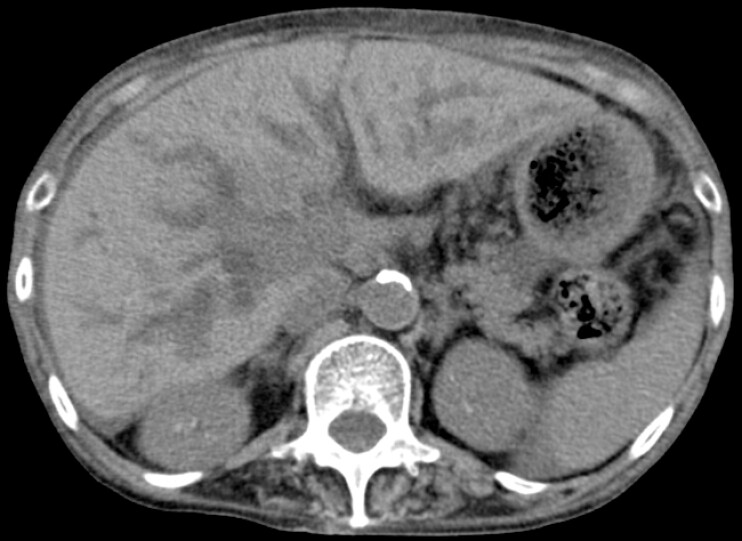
Computed tomography before endoscopic treatment. Dilated intrahepatic bile ducts due to recurrent pancreatic cancer after pancreaticoduodenectomy.
